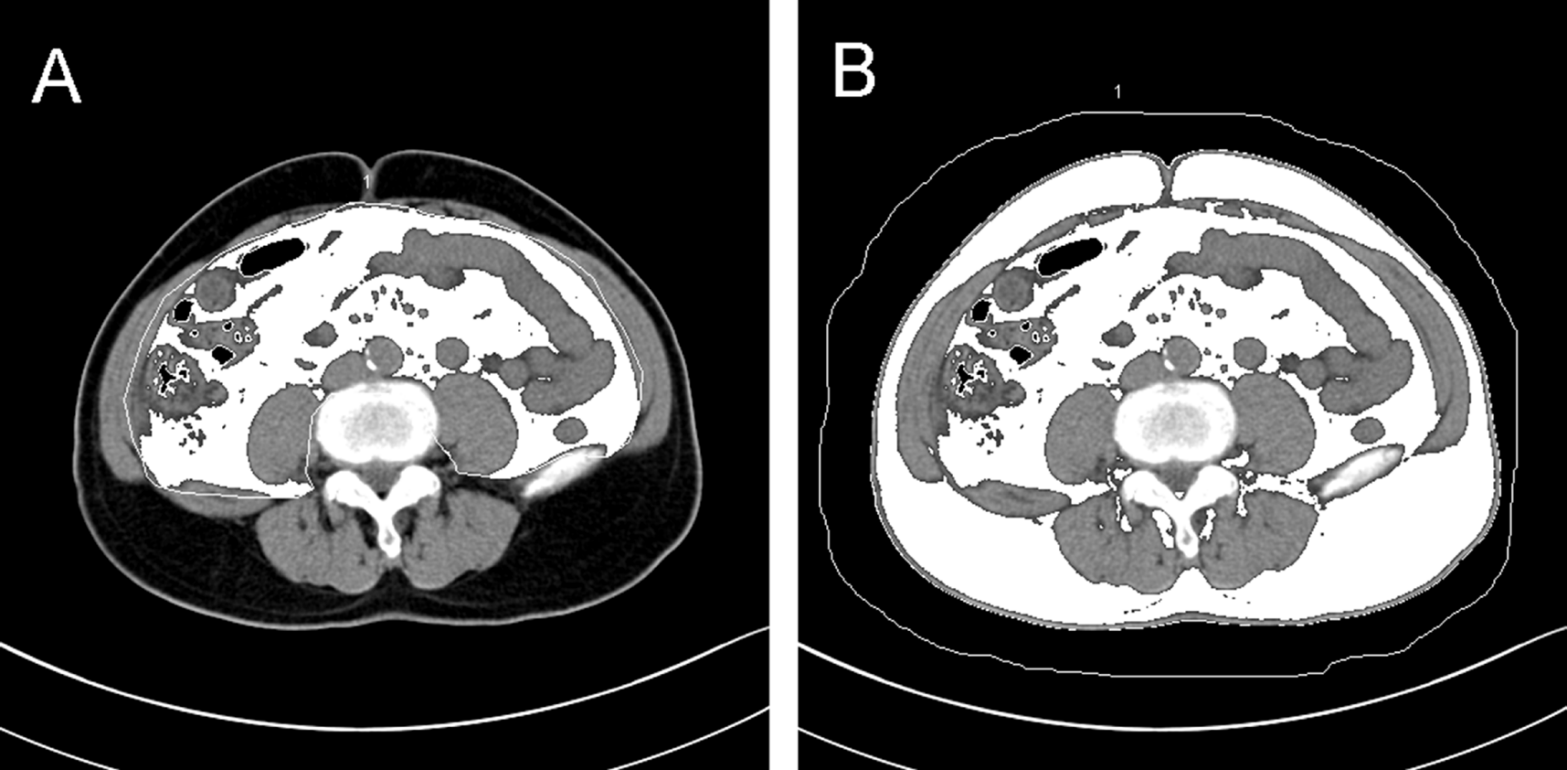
Representative axial CT images at the level of umbilicus showing (A) visceral fat area and (B) subcutaneous fat area plus visceral fat area.
